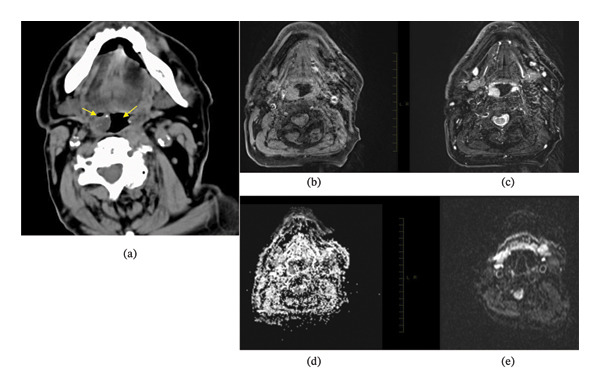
Radiological findings of the palatine tonsillar cysts. (a) Axial computed tomography at the oropharyngeal level demonstrates bilateral, spherical soft‐tissue masses protruding into the oropharyngeal lumen. (b) Axial T1‐weighted magnetic resonance image shows the lesions with an isointense signal to the surrounding soft tissue. (c) Axial T2‐weighted magnetic resonance image reveals high signal intensity of the lesions, consistent with cystic contents. (d) Apparent diffusion coefficient (ADC) map shows no high signal intensity. (e) Diffusion‐weighted imaging demonstrates the absence of restricted diffusion. The masses identified on computed tomography appeared isointense on T1‐weighted images and hyperintense on T2‐weighted images, without hyperintense signals on diffusion‐weighted images or the ADC map.
